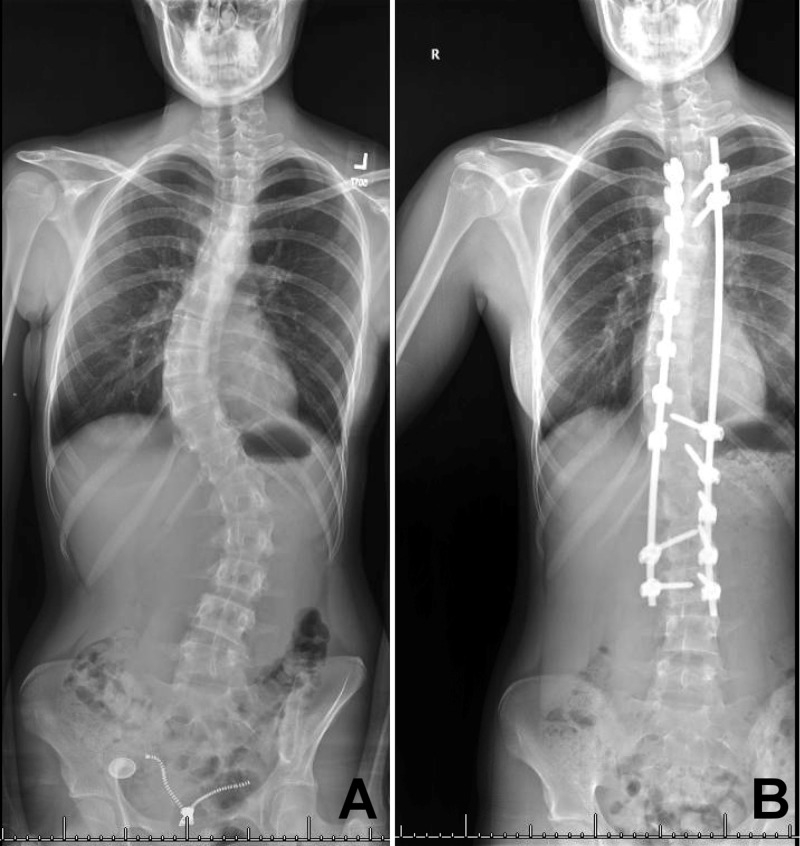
Preoperative (A) and postoperative (B) anteroposterior scoliosis radiographs
